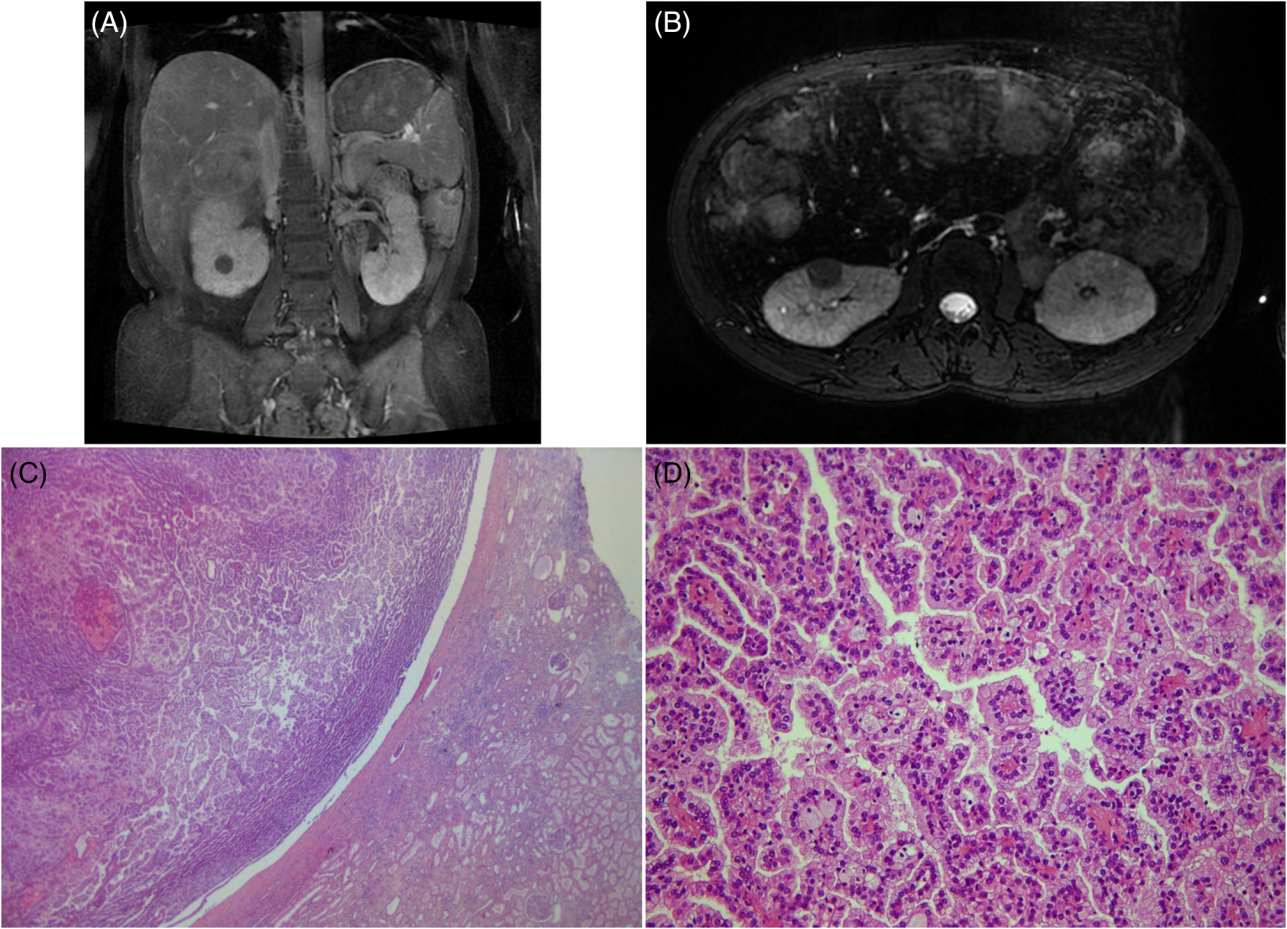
A, Renal MRI, right renal hypo T2 mass (diameter 16 mm). B, Renal MRI, right renal anterior hilar lip hypo T2 and poorly vascularized tumor (diameter 16 mm). C, Papillary renal tumor, HES ×25. D, Papillary renal cell carcinoma type 1, HES ×200
